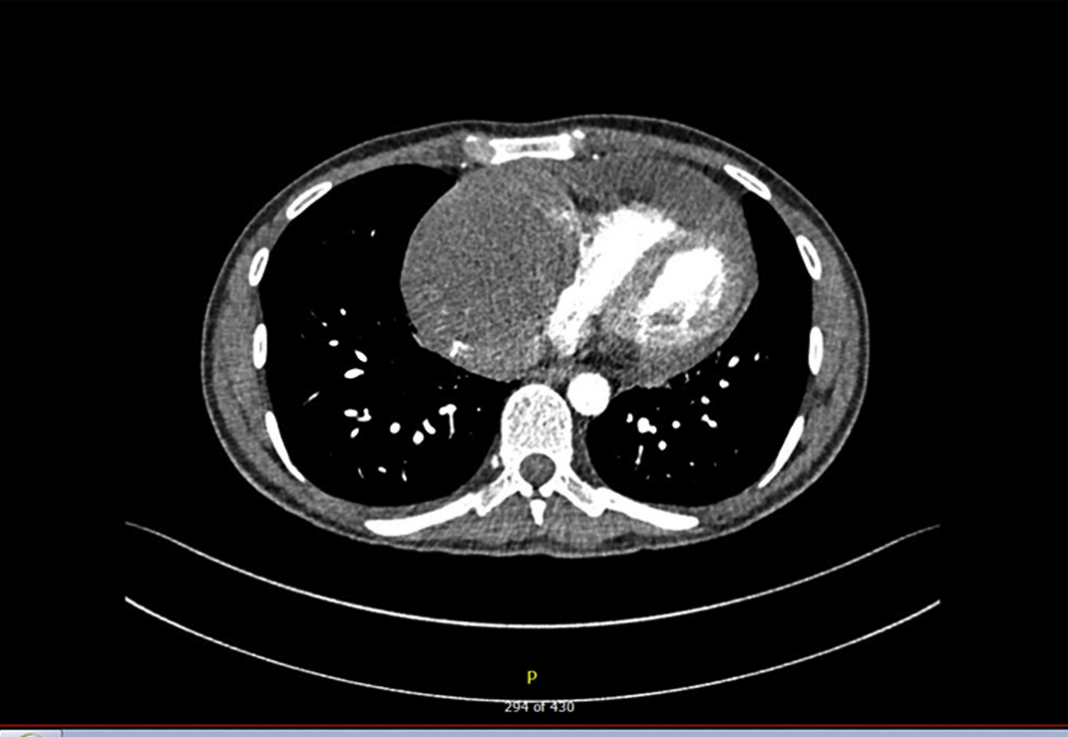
Computed Tomography Pulmonary Angiogram Showing an Anterior Mediastinal Mass
